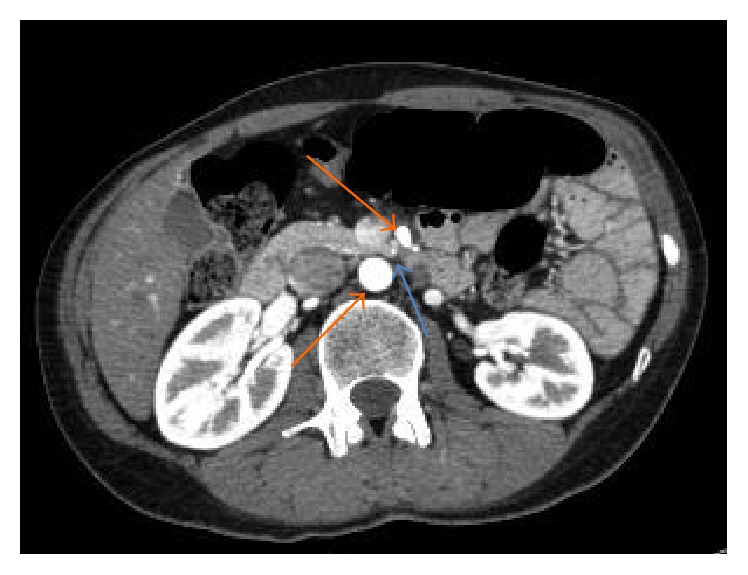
CT scan (axial view) with duodenal compression (blue arrow) between the aorta and superior mesenteric artery (orange arrows) (Case Report One).
